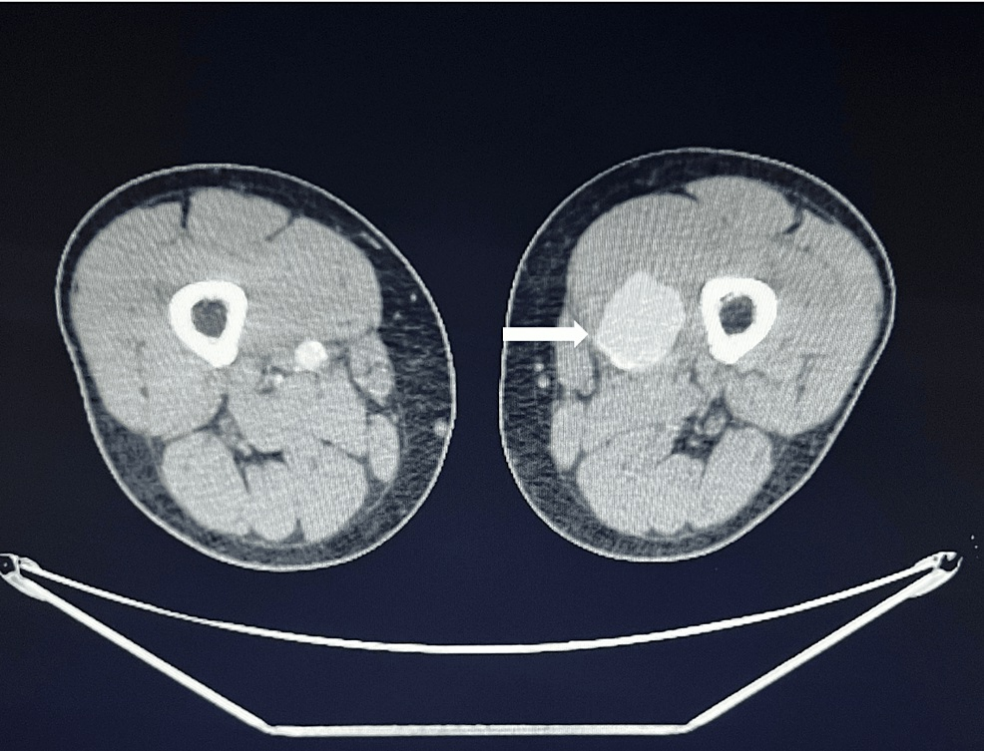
CTA revealing the left-sided superficial femoral artery aneurysm with dimensions of 4x4cm. CTA: Computed tomography angiography
